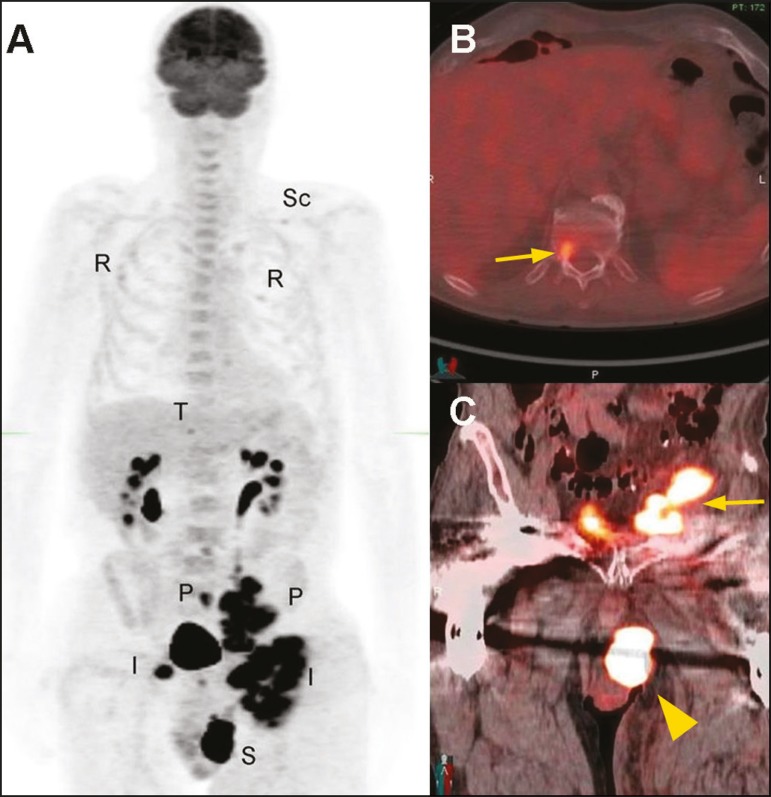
Maximum-intensity projection ^18^F-FDG PET/CT (A)
